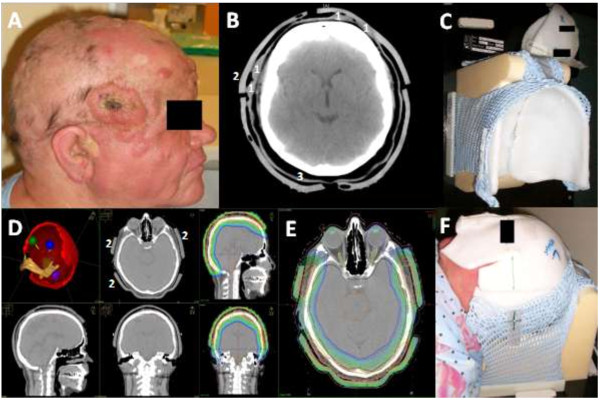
Clinical presentation, treatment planning and setup in patient 1. (A) Clinical presentation of patient 1 with cutaneous manifestation of T-cell lymphoma (mycosis fungoides). (B) Pre-treatment transversal CT scan with nodular scalp lesions (treatment planning CT with bolus in place). (C) Immobilization mask with integrated wax bolus. (D) Contured target volume, organs at risk (green/blue: eye bulb; red PTV scalp; yellow: optic nerves; purple: left parotid; orange: brain stem; beige: mandible bone), bolus and tumor lesion. (E) transversal cranial treatment planning CT with dose distribution over PTV from 12% isodose (red line) to 52% isodose (dark blue line). (1) tumor lesions, (2) bolus, (3) immobilization mask. (F) Complete treatment setup with correct patient positioning, wax bolus and opened immobilization mask.
